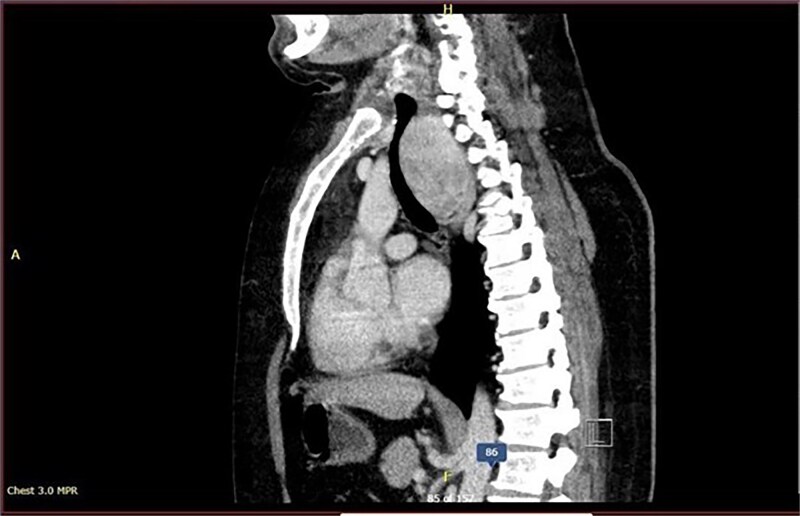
Sagittal CT image demonstrate a posterior mediastinal mass with significant compression and displacement of adjacent structures.
